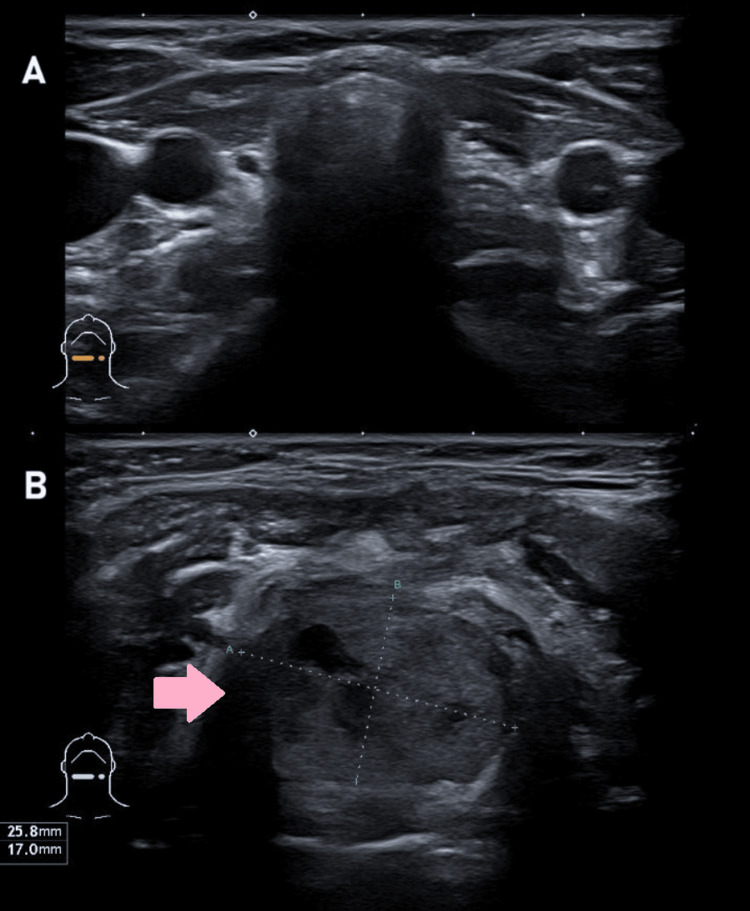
Last anterior neck ultrasound (transverse view). (A) Absence of the thyroid gland in its usual topography (B) A predominantly solid nodular formation in the submental region (pink arrow), 26x17 mm
